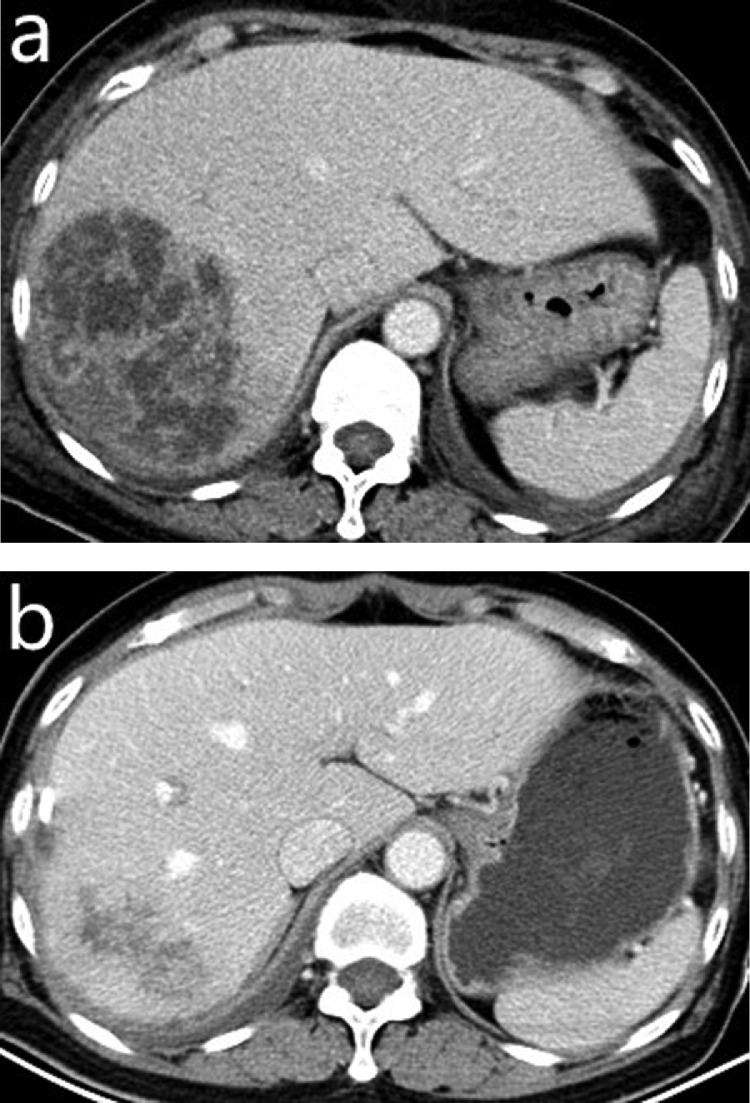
In a 56-year-old liver abscess male with diabetes mellitus, the contrast-enhanced portal-venous phase CT images show the preoperative liver abscess cavity (a), and the obvious shrinkage of it (b) and no *pus* can be drained 32 days after the CT-guided interventional therapy.
